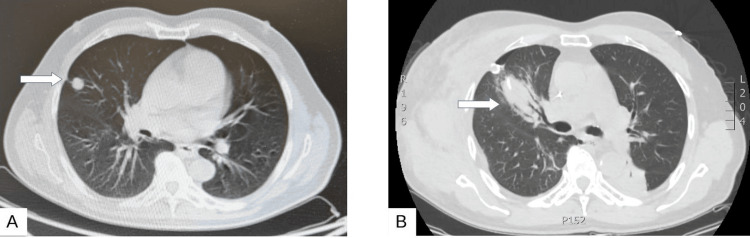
Preoperative CT scan. A) The atypical carcinoid is presented as a nodule in the lateral segment of the middle lobe (arrow). B) Postoperative CT scan. A chest tube and a small apex pneumothorax can be seen, along with lung consolidation of the right upper lobe (arrow) and a chest wall hematoma.
